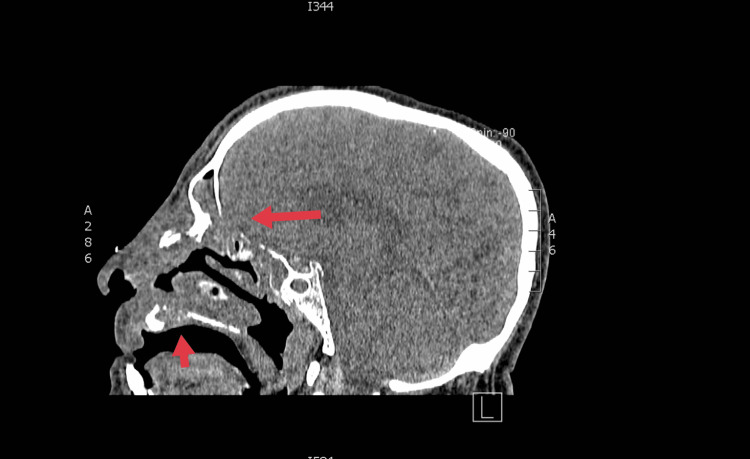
Sagittal plane: Cranial computed tomography scan. Arrows indicate the osteolytic regions of the hard palate and posterior table of the frontal sinus.
